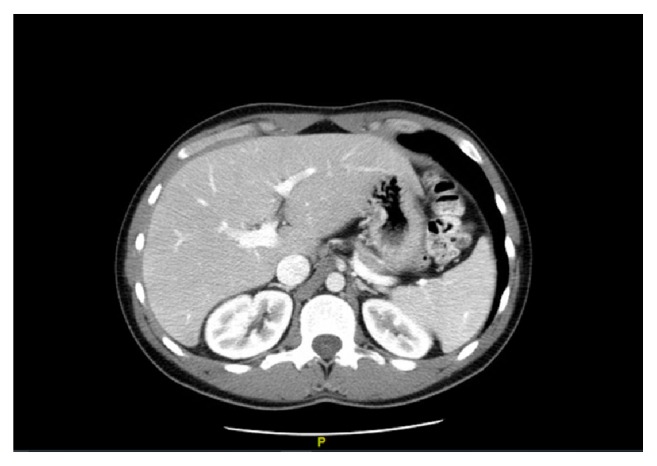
An axial CT imaging of the abdomen showing resolution of pyogenic liver abscess in this patient.
